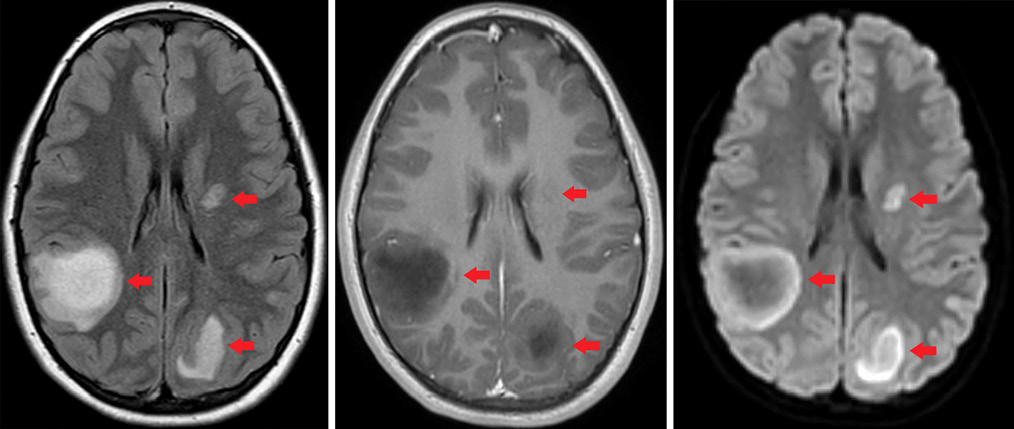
Initial brain MRI findings. Left: Axial FLAIR image demonstrating multifocal supratentorial subcortical and deep white matter lesions (red arrows). Middle: Axial T1 post-contrast image demonstrating peripheral lesion enhancement. Right: Axial DWI image demonstrating avid peripheral diffusion restriction. DWI, diffusion-weighted imaging; FLAIR, fluid attenuation inversion recovery.
